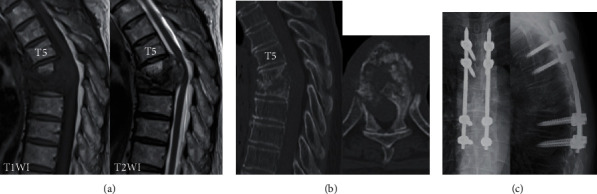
Imaging findings at the initial visit. (a) MRI sagittal imaging. T1 low and T2 iso-to-low extradural mass formation and severe compression to the spinal cord were observed. (b) CT sagittal reconstruction and axial imaging at T6. Osteolytic destruction of T6 and T7 vertebrae was observed. (c) X-ray just after the primary surgery.
